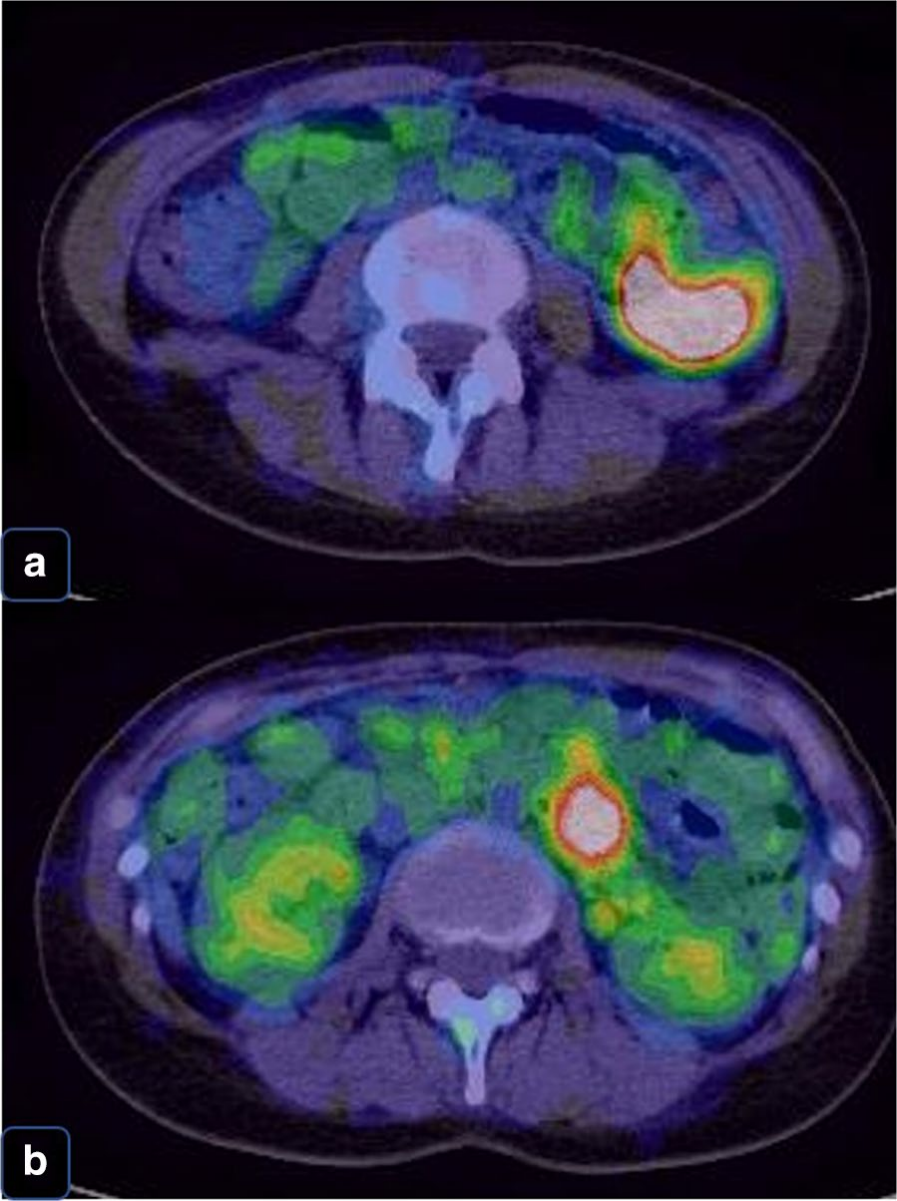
PET–CT confirmed an abnormal accumulation of F-18 fluorodeoxyglucose in the tumor and lymph nodes. a Primary tumor’s accumulation. b Lymph nodule’s accumulation
